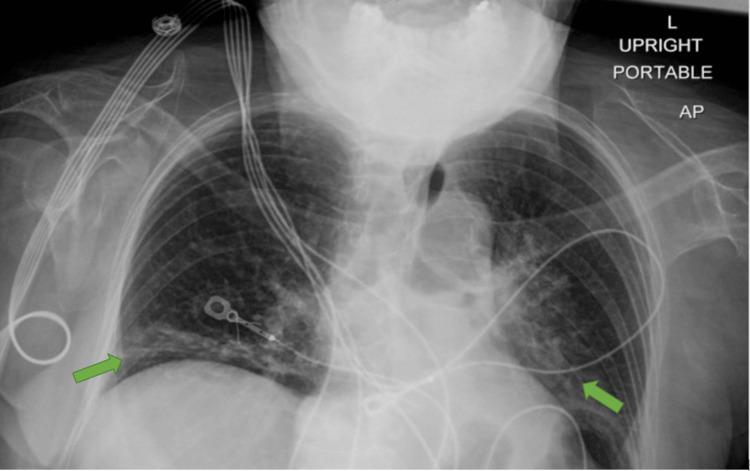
X-ray indicating bibasal atelectasis. Green arrows indicate areas of bilateral atelectasis along the diaphragmatic margins.
